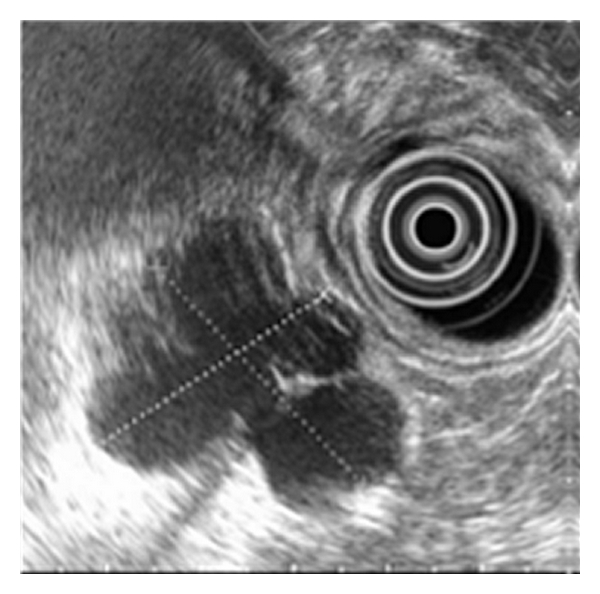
Endoscopic ultrasound image showing the cyst in pancreatic body.
